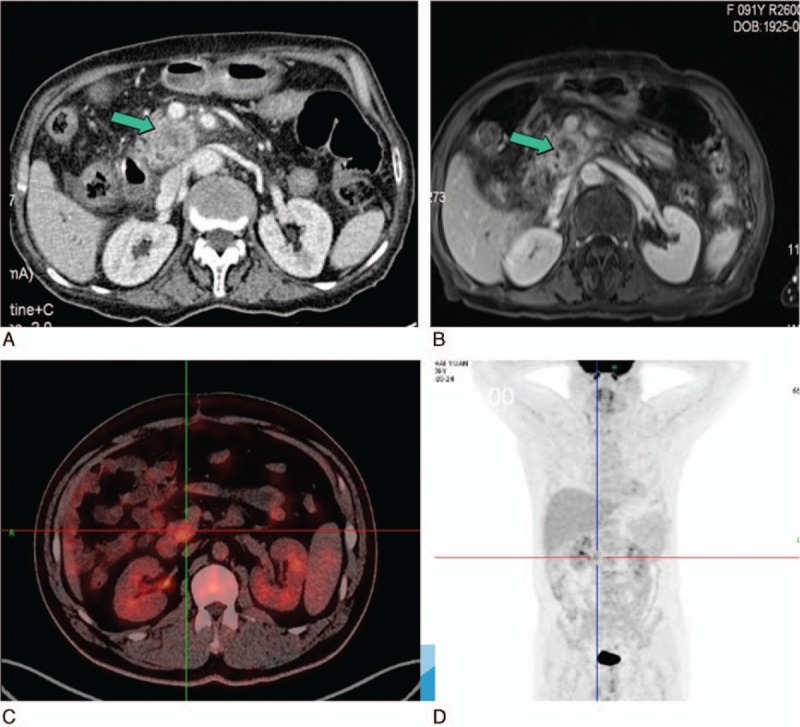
(A) Abdominal contrast-enhanced CT and (B) contrast-enhanced MRI showed a 3 × 3 cm solid hypovascular tumor in the head of the pancreas (green arrow). (C, D) PET-CT showed a mass with SUVmax 11.6 located in the pancreatic head and no distant organ metastasis. CT = computed tomography, MRI = magnetic imaging resonance, PET = positron emission tomography.
